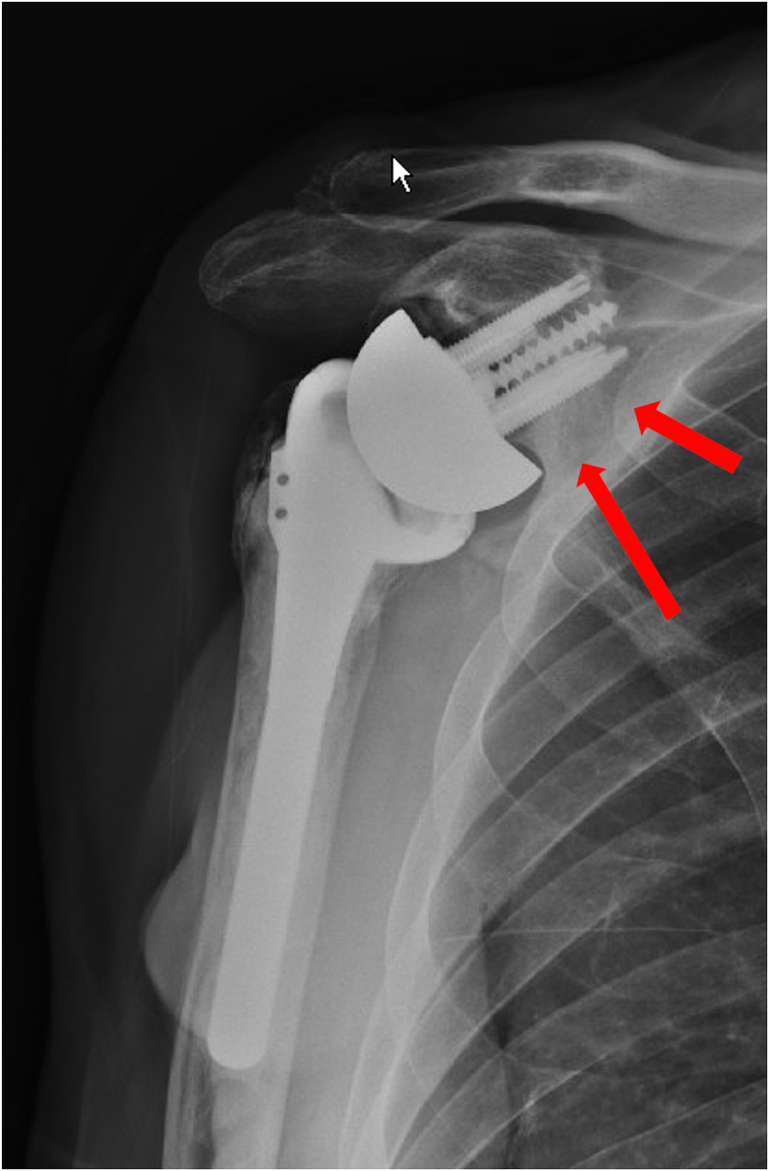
X-ray Grashey view demonstrating a healed fracture at the scapular body.
